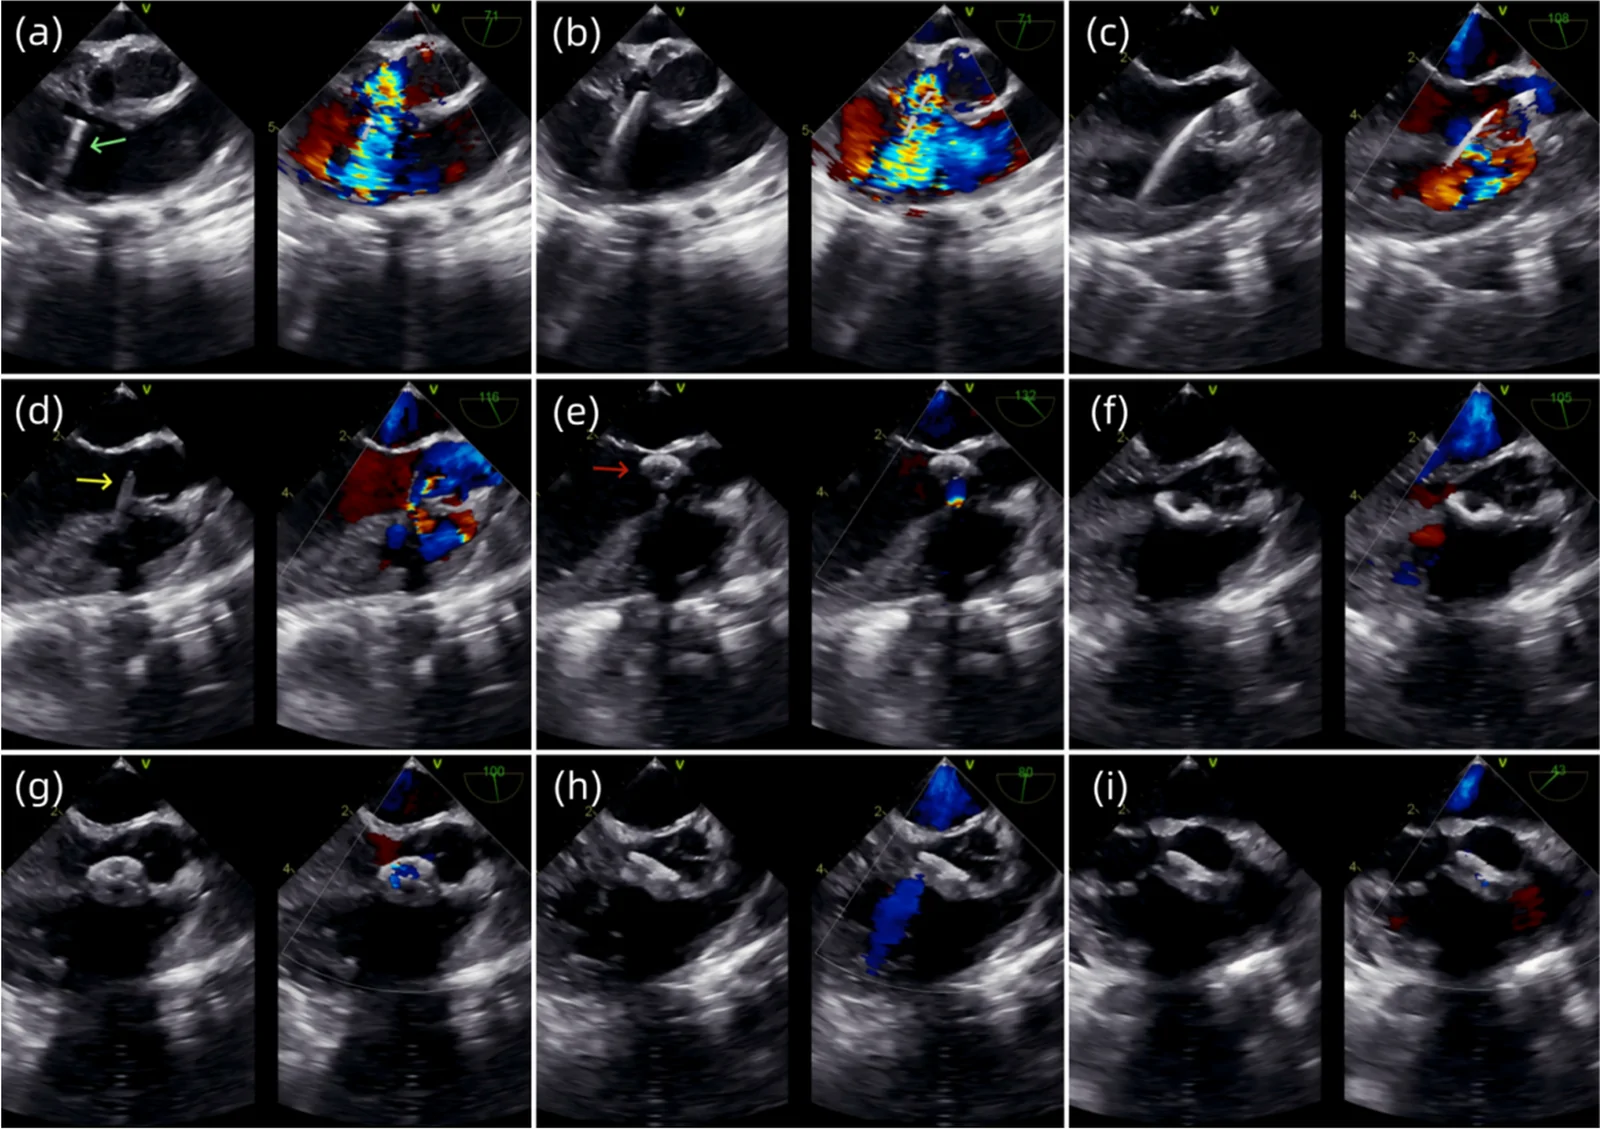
Transthoracic implantation of the bioabsorbable occluder under TEE guidance. (a–i): (a) RV free-wall puncture and guidewire (green arrow) advancement. (b) Guidewire crossing the pmVSD from the RV side. (c) Guidewire tip positioned in the ascending aorta (parasternal long-axis view). (d) Delivery sheath (yellow arrow) advanced into the LV. (e) Left disc deployment (red arrow). (f) Left disc retracted against the septum. (g) Waist and right disc deployment. (h) Final locked occluder with no AR. (i) No TR on short-axis view. LV, left ventricle; RV, right ventricle; AR, aortic regurgitation; TR, tricuspid regurgitation; pmVSD, perimembranous ventricular septal defect.
